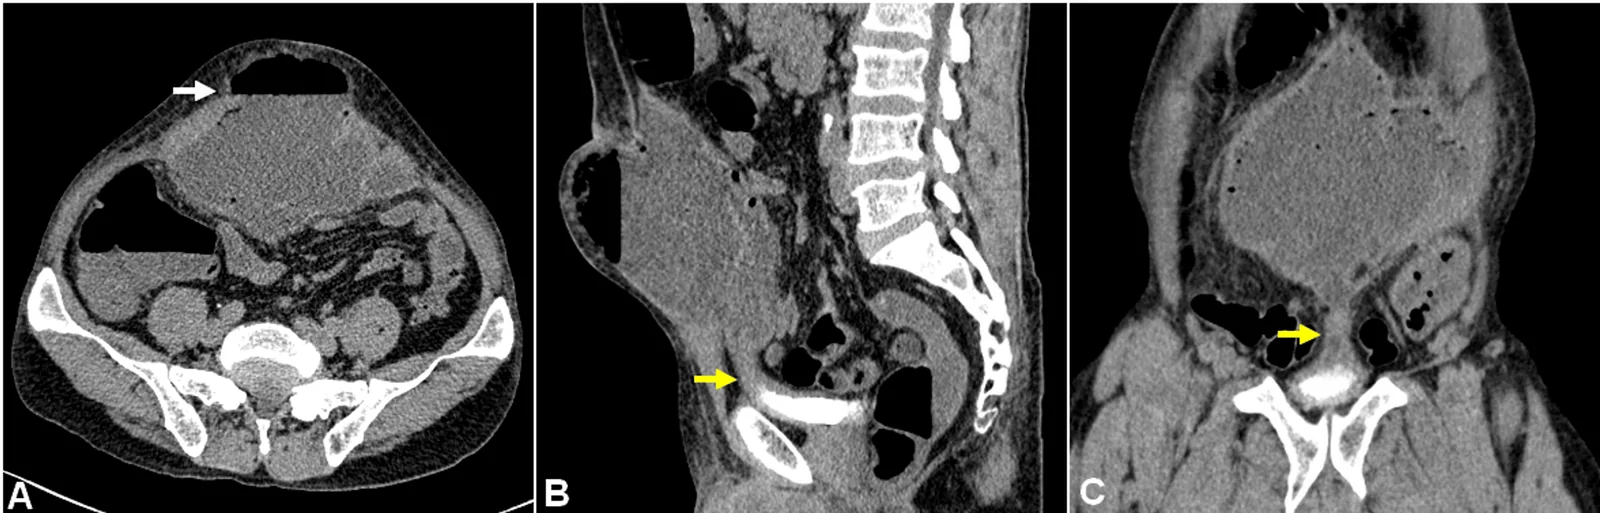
Abdominal contrast-enhanced CT scan image showing a large urachal abscess (A) Axial image showing a thick-walled heterogeneous cystic mass in the preperitoneal space containing a gas-fluid level (white arrow). (B) Coronal and (C) sagittal images showing a preperitoneal cystic mass below the umbilicus, with a cord-like structure that extends to the bladder (yellow arrows), but no evidence of communication between the mass and the bladder.
